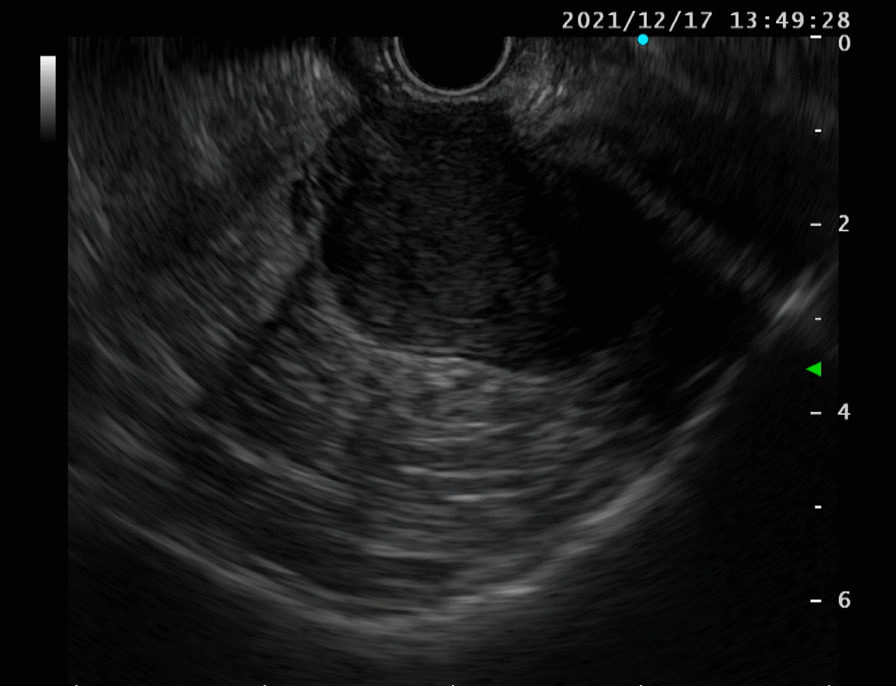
Endoscopic ultrasound-guided fine-needle aspiration. A borderline oligo-hypoechoic mass in the splenic hilum
